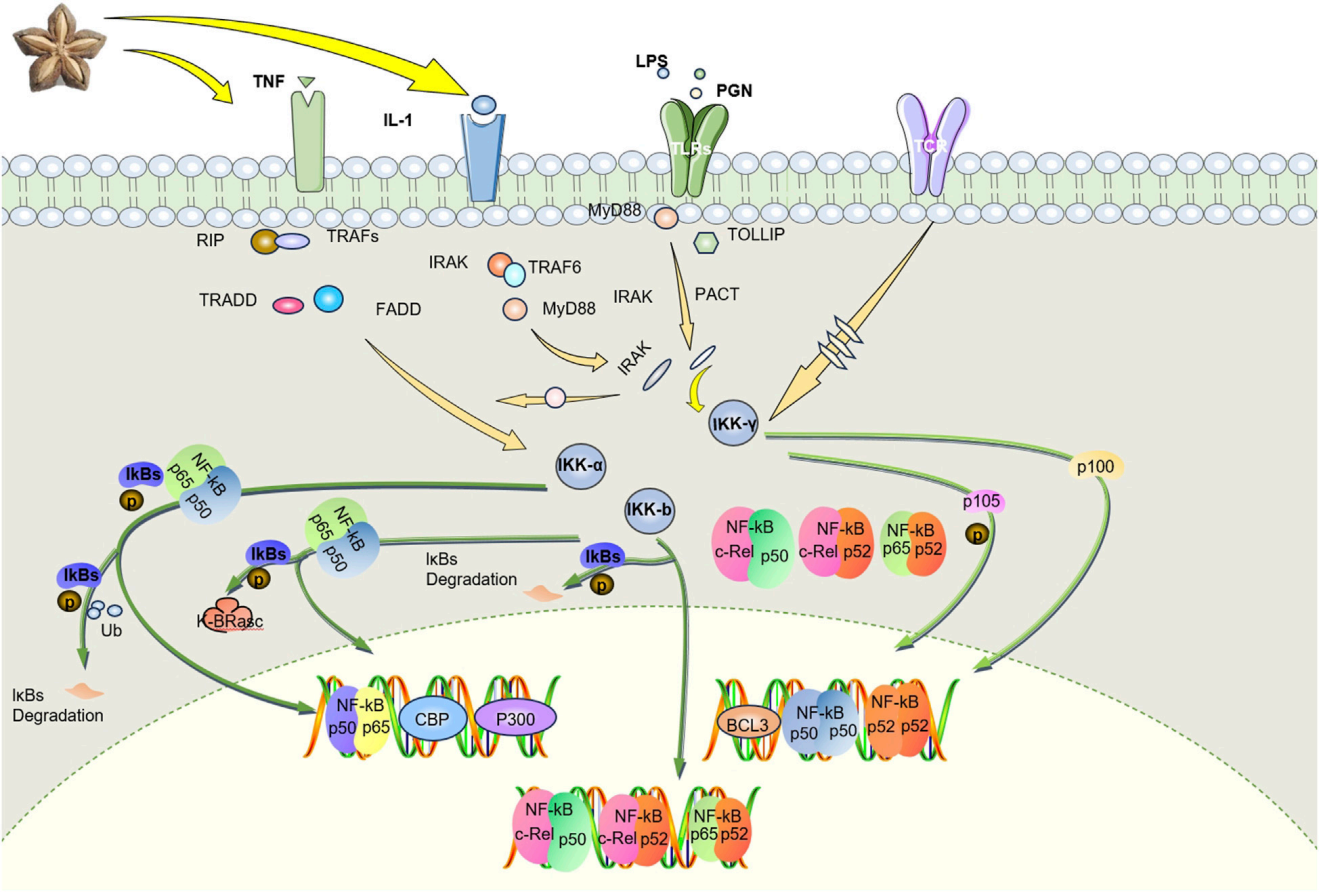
SI targets TNF- α mediate NF-κB pathway exerts anti-RA effects. SI inhibits the proliferation of macrophage by negatively regulating the NF-κB pathway and promotes their apoptosis by playing an anti-inflammatory role. IL-1, interleukin-1; TNF, tumor necrosis factor; LPS, lipopolysaccharide; PGN, peptidoglycan; TLRs, toll like receptors; TCR, T cell receptor; RIP, receptor-interacting protein; TRAFs, tumor necrosis factor receptor associated factors; TRADD, tumor necrosis factor receptor 1 associated death domain protein; FADD, fas-associating protein with a novel death domain; IRAK, interleukin-1 receptor associated kinase; TRAF6, tumor necrosis factor receptor associated factor 6; MyD88, myeloid differentiation primary response gene (88); PACT, photoacoustic computed tomography; TOLLIP, toll-interacting protein; Ikk-α, inhibitor of nuclear factor-κB kinase-α; Ikk-β, inhibitor of nuclear factor-κB kinase-β; Ikk-γ, inhibitor of nuclear factor-κB kinase-γ; IKBs, inhibitor of kappa B.
